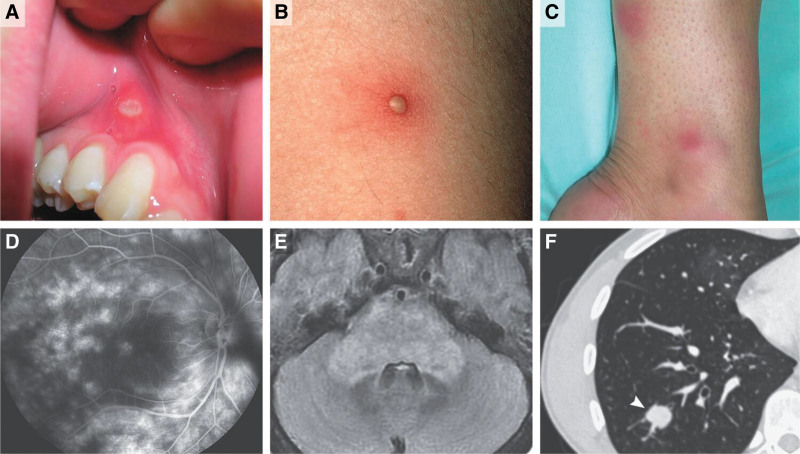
Clinical Manifestations of Behçet’s Syndrome. (A) oral ulcer. (B) a papulopustular lesion. (C) erythema nodosum (EN). (D) capillary impairment and vascular leakage on retinal fluorescein angiography. (E) a brain-stem inflammatory lesion on a T2-weighted axial magnetic resonance imaging sequence, with an edematous, protuberant FLAIR (fluid-attenuated inversion recovery) hypersignal extending to the middle cerebellar peduncles and discrete hypertrophy. (F) aneurysmal dilatation of a pulmonary-artery branch (arrowhead). Saadoun D, et al. Behçet’s Syndrome. N Engl J Med. 2024 Feb 15;390(7):640–651. doi: 10.1056/NEJMra2305712. PMID: 38354143.*.*With permission from the New England Journal of Medicine (NEJM), License Number 6075080861718.
